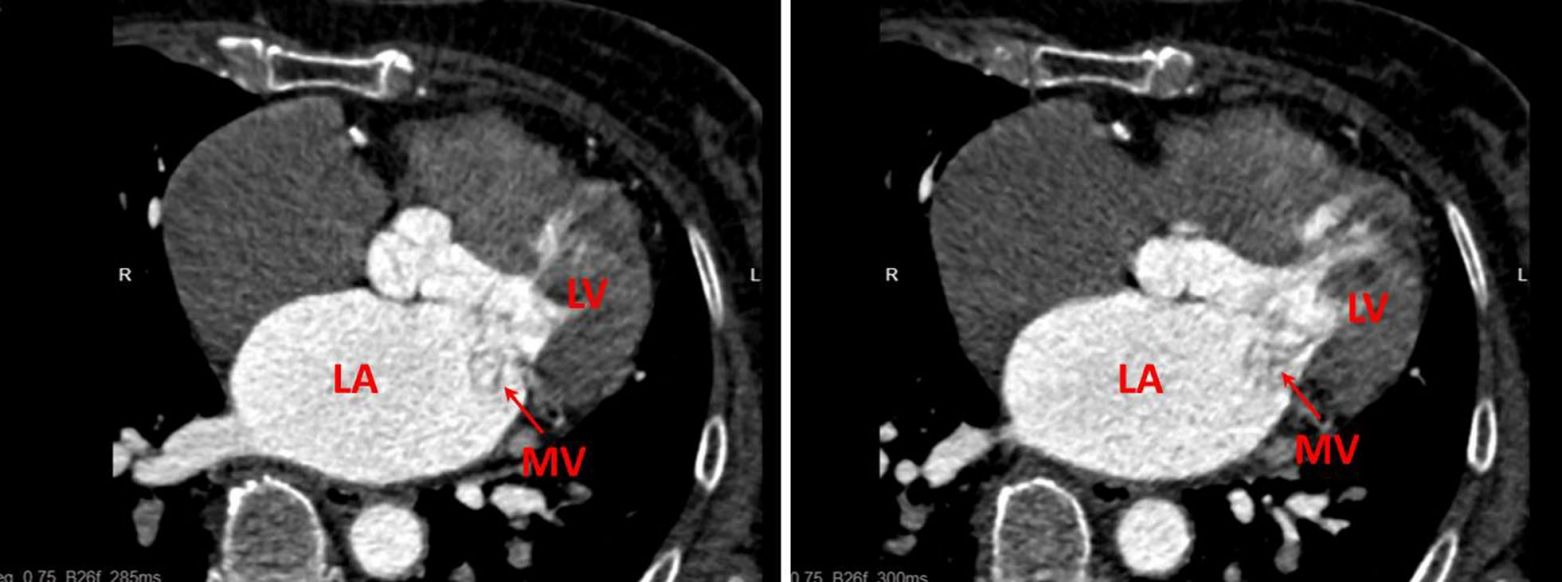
Coronary computed tomography angiography showed polycystic structures at the position of mitral valve
